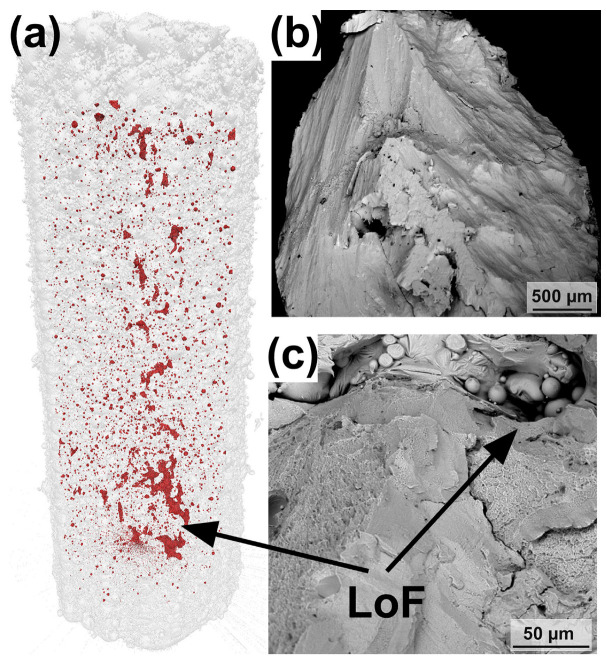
(a) The μ-CT of the LPBF-fabricated Zr-based BMG compression sample. (b,c) Fracture surfaces of the LPBF-fabricated BMG sample. Two intersecting fracture surfaces can be seen in (b), and unmelted powder particles were found in some larger LoFs in (c) [40].
